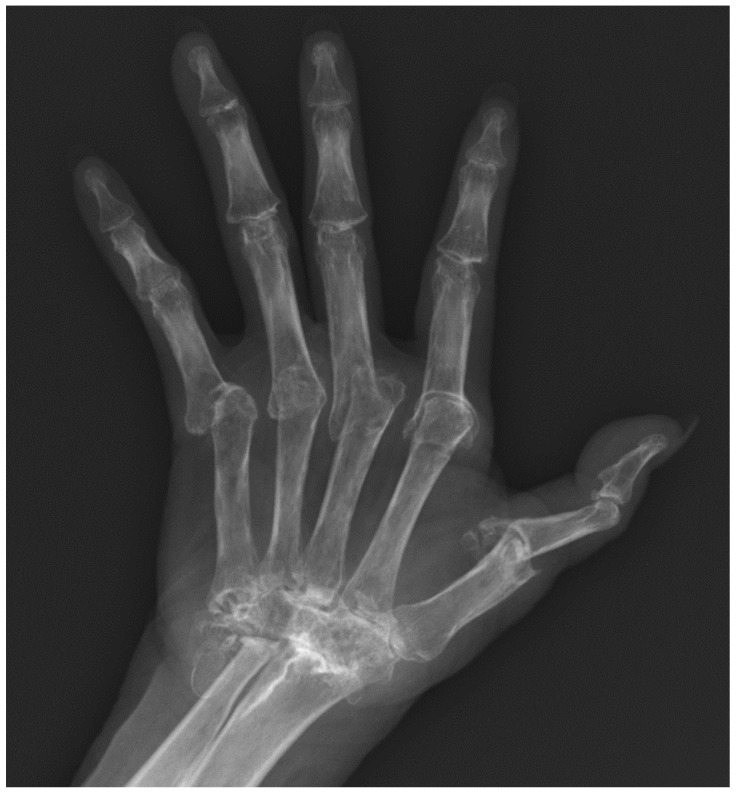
59-year-old female rheumatoid arthritis. Hand radiograph shows extensive erosive changes and osseous loss in the wrist and metacarpophalangeal joints with telescoping and ulnar deviation at the MCP joints. Severe demineralization is also present. Findings represent arthritis mutilans which also can be seen in psoriatic arthritis.
